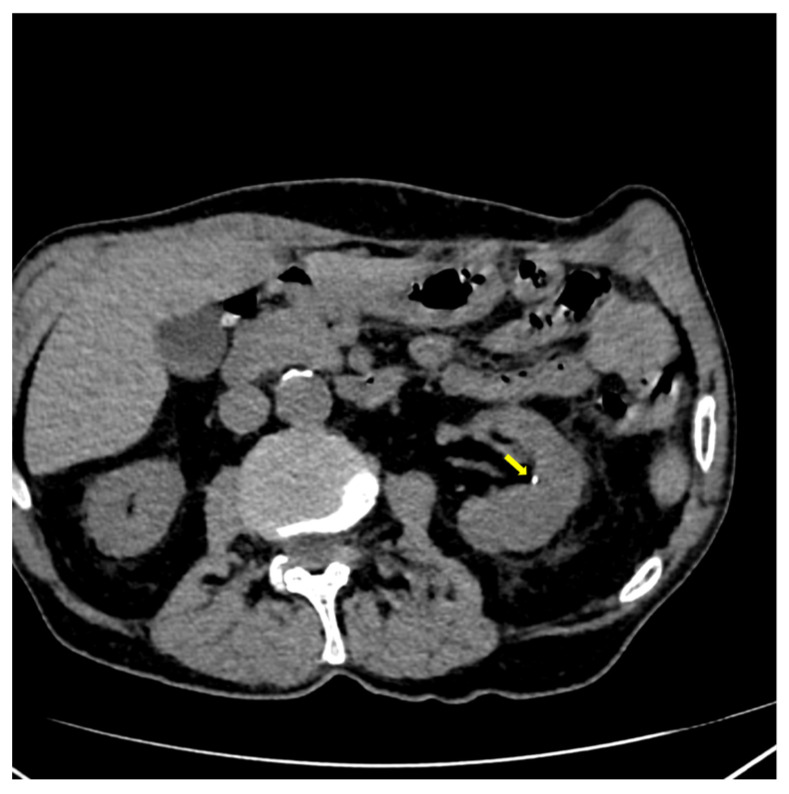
CT showing micro-calculi (yellow arrow) in the middle calyx of the left kidney.
